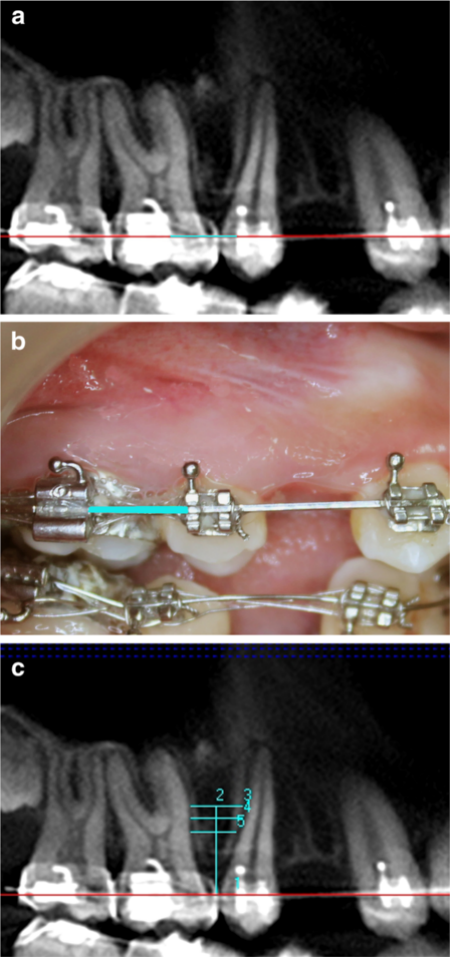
Orthodontic arch wire in second premolar and first molar region taken as standard reference for measurements. (a) Panorex View of CBCT image, (b) correlated clinically, and (c) various heights from the arch wire within the limits of mucogingival junction selected for bone measurements.
